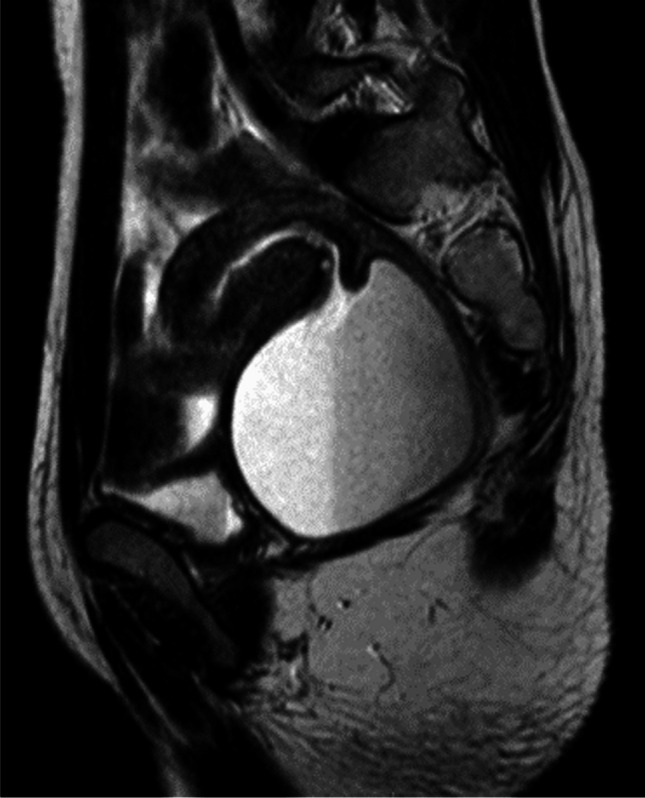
Magnetic resonance imaging showing haematocolpos in the proximal vagina
